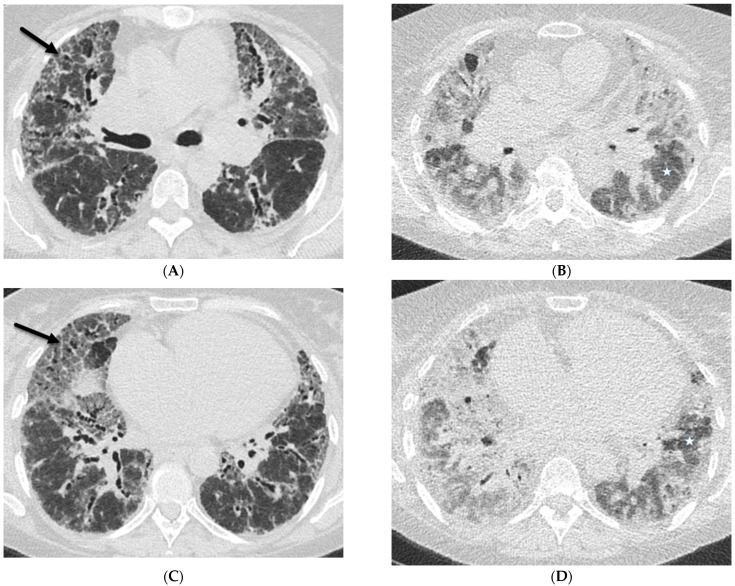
A 60-year-old female with a history of chronic hypersensitivity pneumonitis presents with worsening hypoxemia. Axial inspiratory (A,C) and expiratory (B,D) chest CT images demonstrate areas of interlobular septal thickening (solid black arrows), ground glass opacity, and traction bronchiectasis. There is also heterogeneous pulmonary air trapping on the expiratory images (stars).
